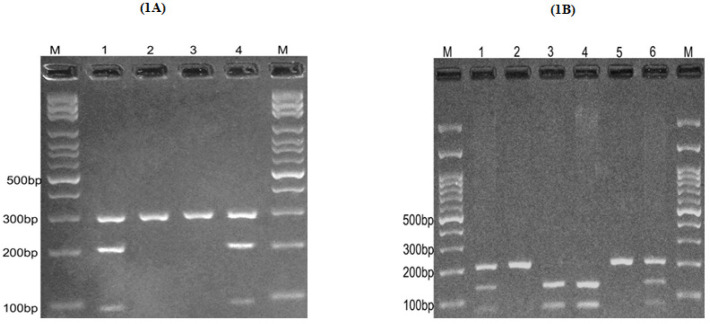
(1A) electrophoresis pattern of the PCR-RFLP method for the detection of *PD-1*rs11568821(G/A) polymorphism. M: DNA marker; Lanes 1 and 4: GA; Lanes 2 and 3: GG (1B): Photograph of electrophoresis pattern of the PCR-RFLP method for detection of *PD-1* rs2227981(C/T) polymorphism. M, DNA marker; Lanes 1 and 6, CT; Lanes 2 and 5, CC; lanes 3 and 4, TT
